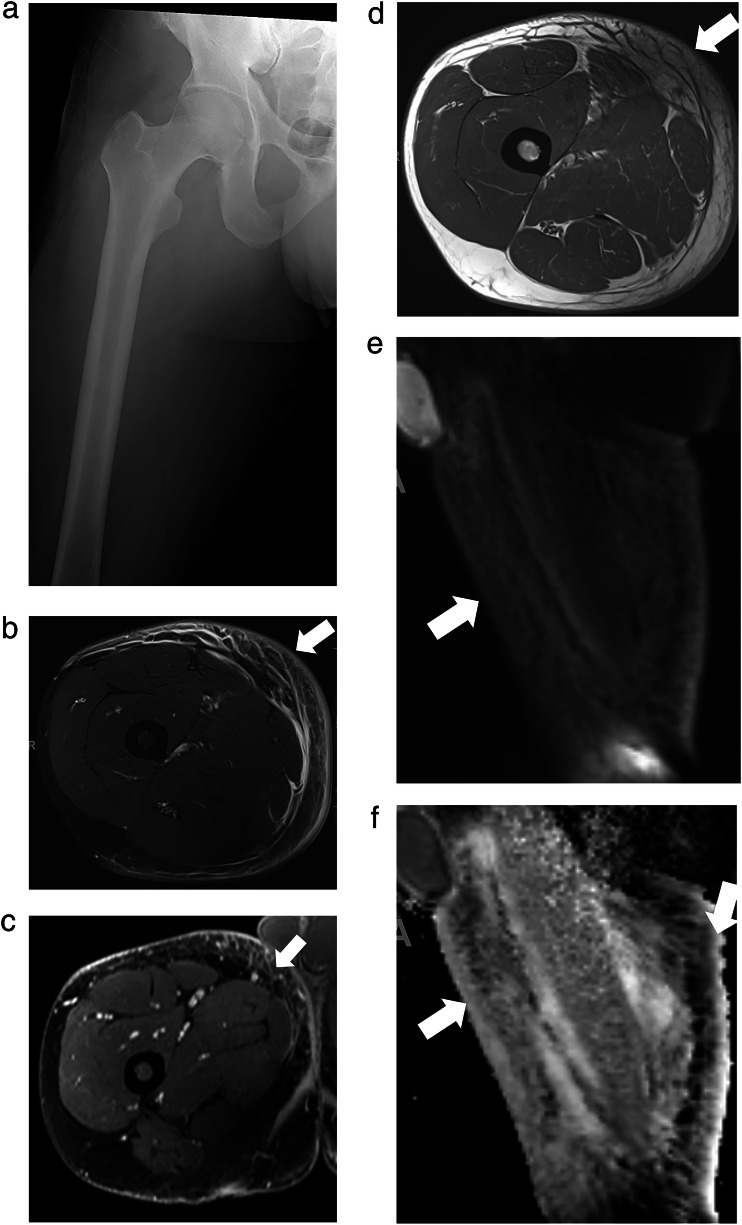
MSKI-RADS Class II. A 35-year-old man with right thigh pain and swelling. a–d Frontal X-ray, Axial T1W and fsT2W (water map Dixon), and axial 3D fsT1W post-contrast images, respectively, of right thigh showing soft tissue edema, skin thickening and cellulitis (arrows) consistent with superficial soft tissue infection (class II). e, f Corresponding DWI and ADC sagittal maps confirm cellulitis with no significant diffusion restriction to suggest underlying abscess
